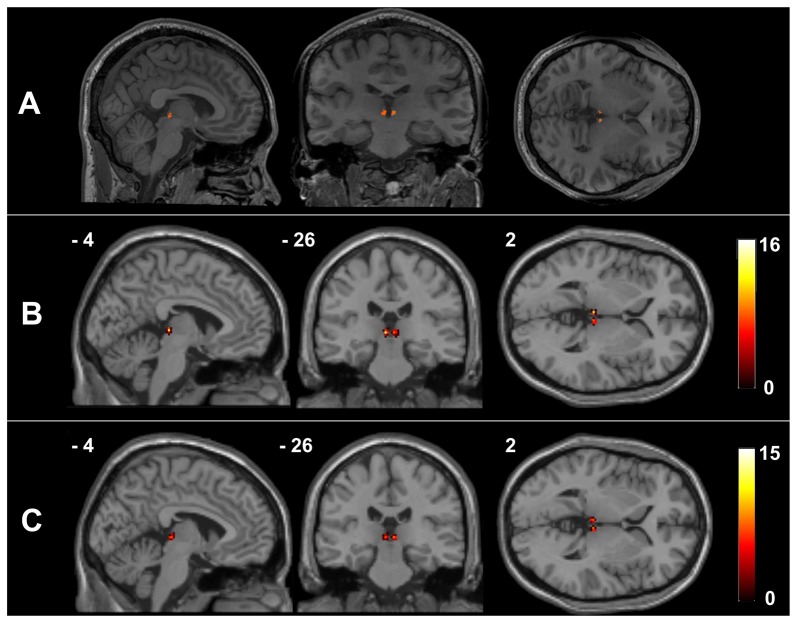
The habenula regions of interest (ROIs) in native and Montreal Neurological Institute (MNI) spaces. Individual habenula ROIs were first drawn manually on the high-resolution T1-weighted structural images in native space and are shown for a representative participant (A). The resulting ROIs were then transformed from the native space into the MNI space by applying the deformation fields derived from the tissue segmentation of each participant’s T1-weighted image, after which they were summed over all the healthy controls (HCs) (B) and schizophrenia (SCH) patients (C) to demonstrate the consistency of the habenula locations.
